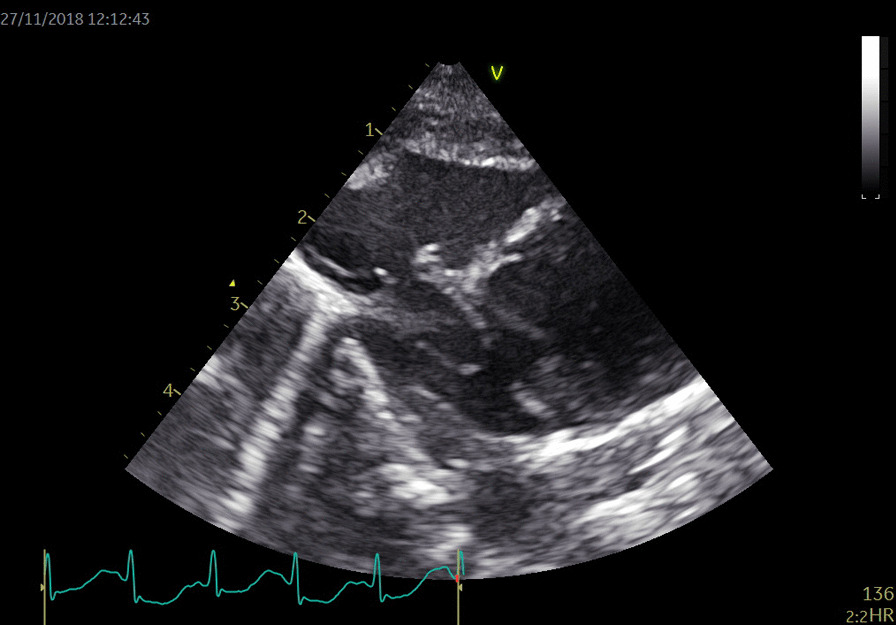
Ultrasound image of the septum with an aneurism of the oval foramen
